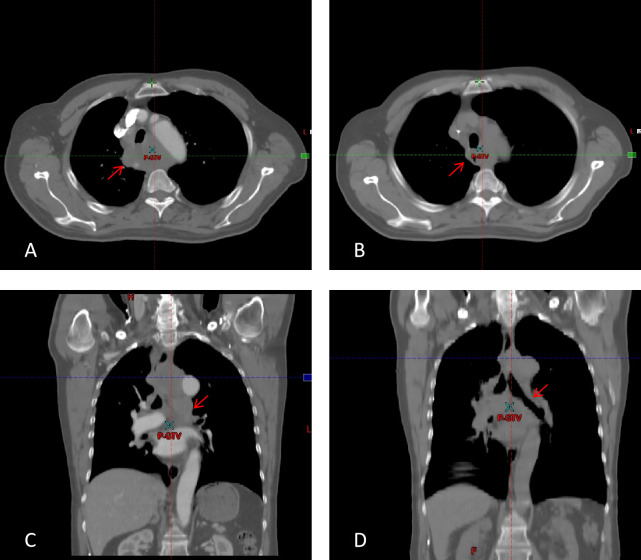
Cross-sectional and coronal CT images before and after treatment. (A, C) axial and coronal images before treatment, respectively. (B, D) axial and coronal images after 5 fractions of SFRT, respectively.
